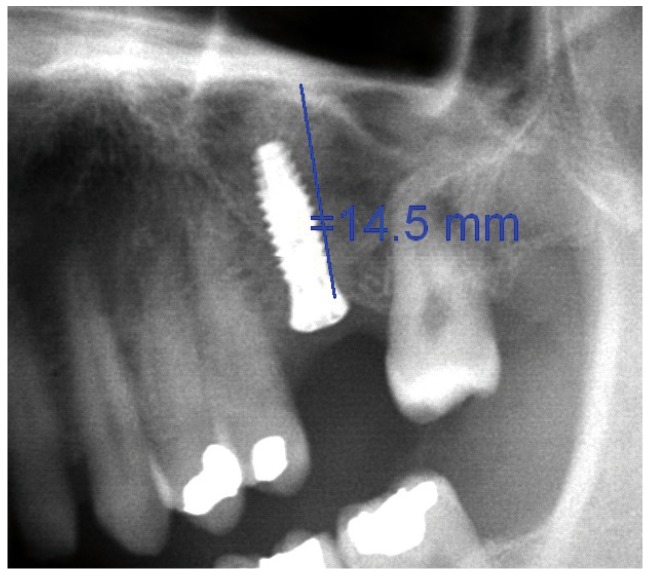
Case 1. 8. Postoperative panoramic X-ray view.
